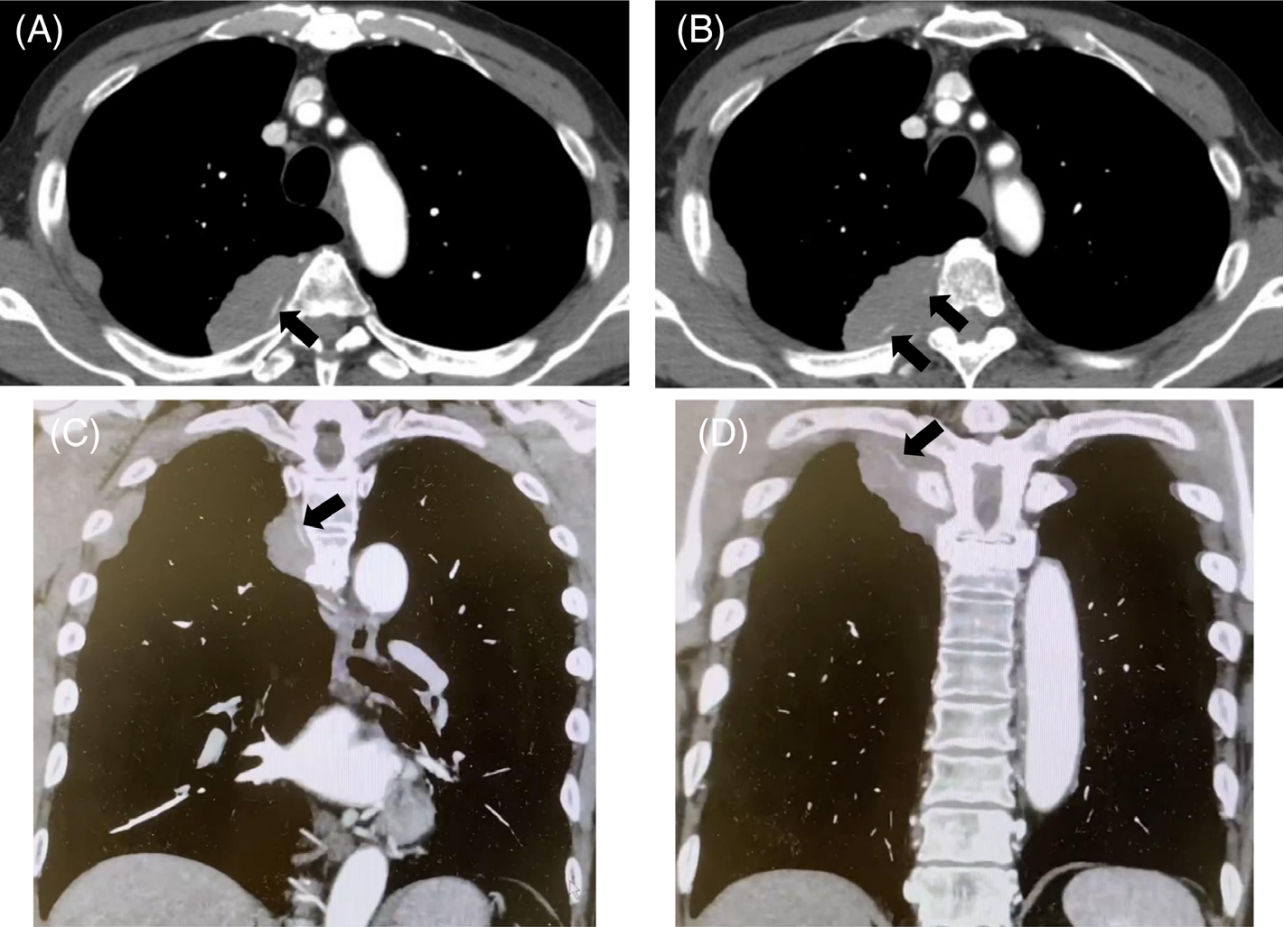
Axial (A, B) and coronal (C, D) CT images showing a right chest wall mass encasing the intercostal arteries, indicative of a positive ‘pleural sandwich sign’ (arrow).
